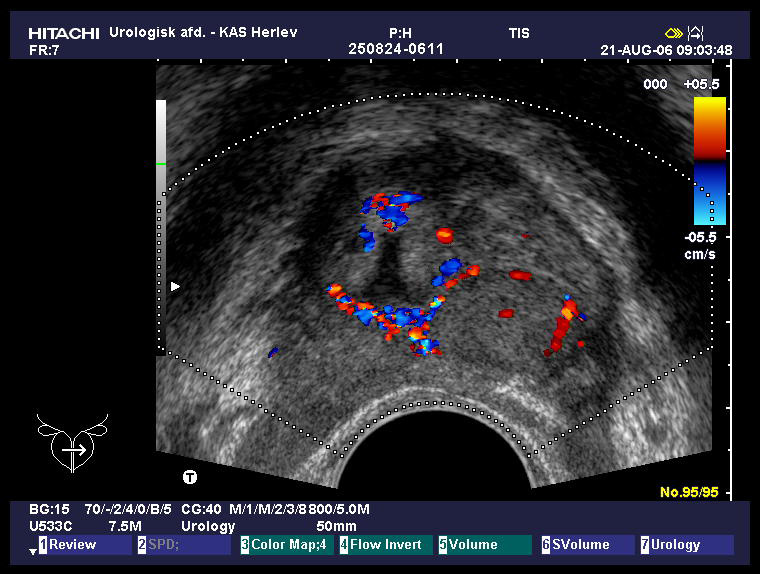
Colour Doppler transrectal ultrasound, transverse image of the prostate. Surrounding the TURP resection cavity tiny blood vessels representing the unresected part of the haemangioma are seen.
